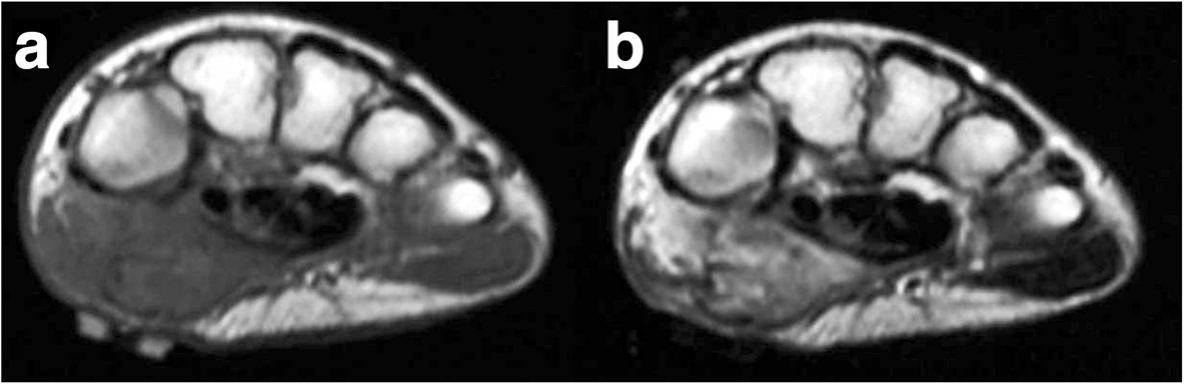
Axial magnetic resonance images of the hand. (a) T1-weighted (repetition time (TR), 561ms; echo time (TE), 20ms) and (b) T2-weighted (TR, 3500ms; TE, 90ms) images of the right hand showing a well-circumscribed mass occupying the thenar muscles.
